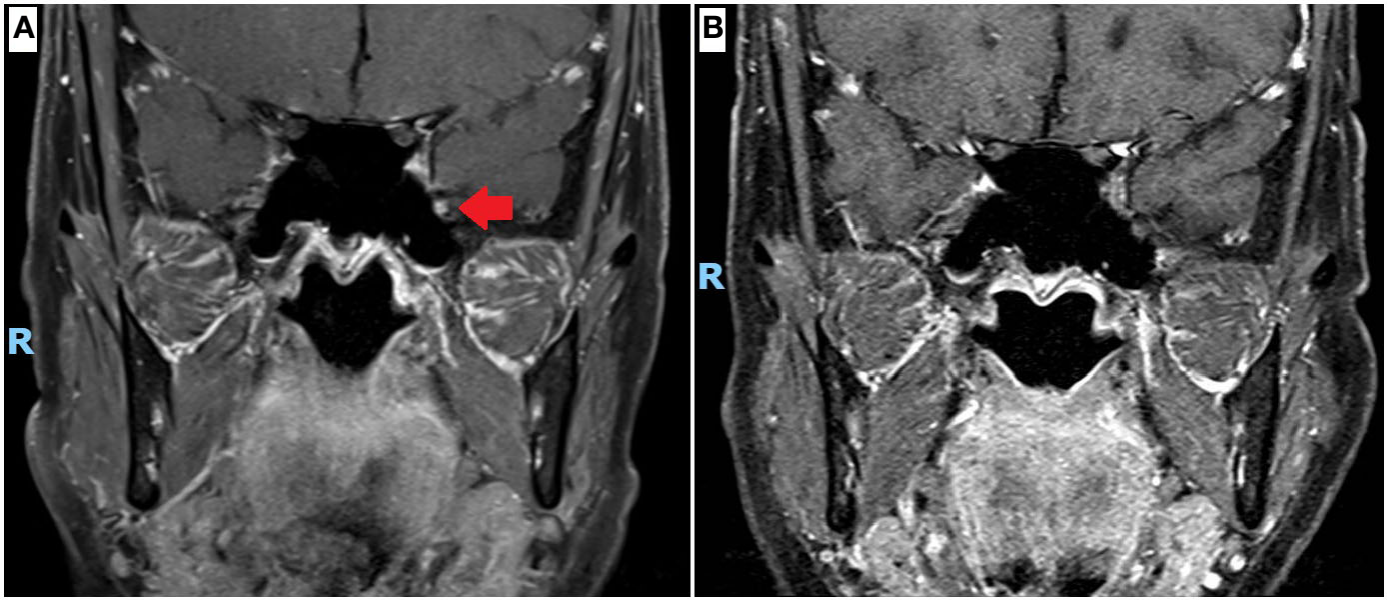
Magnetic resonance imaging (MRI) scan of the cranial nerves. (A) Coronal projection, T1 with gadolinium contrast at 12 months since onset of left numb cheek, showing left maxillary nerve enhancement at the foramen rotundum (red arrow). (B) Coronal projection, T1 with gadolinium contrast at 26 months since onset, performed after completion of neoadjuvant chemotherapy and surgical resection of breast cancer, showing resolution of left maxillary nerve enhancement.
